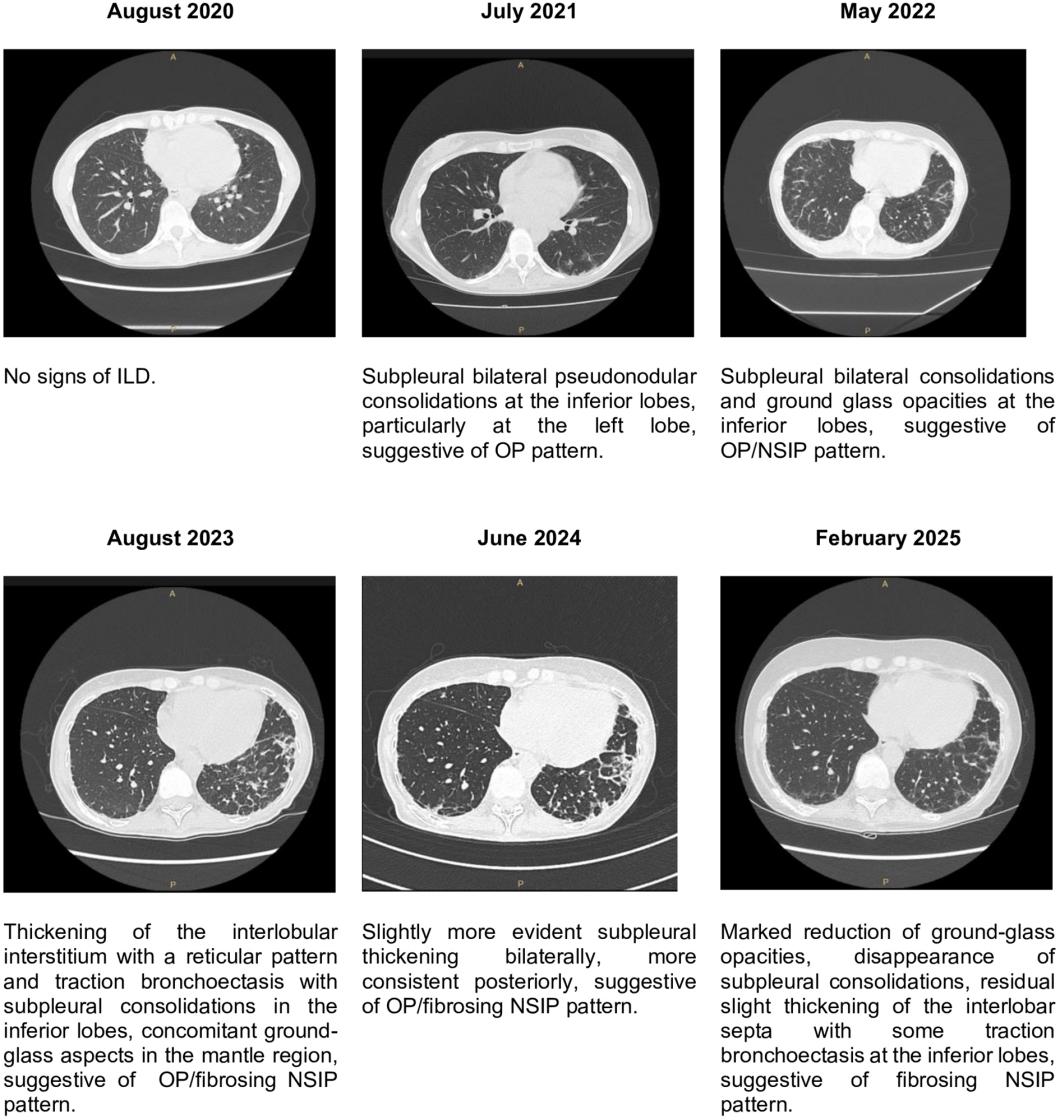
Images and radiological reports of lungs HRCT, showing the more important findings and the predominant ILD pattern.
